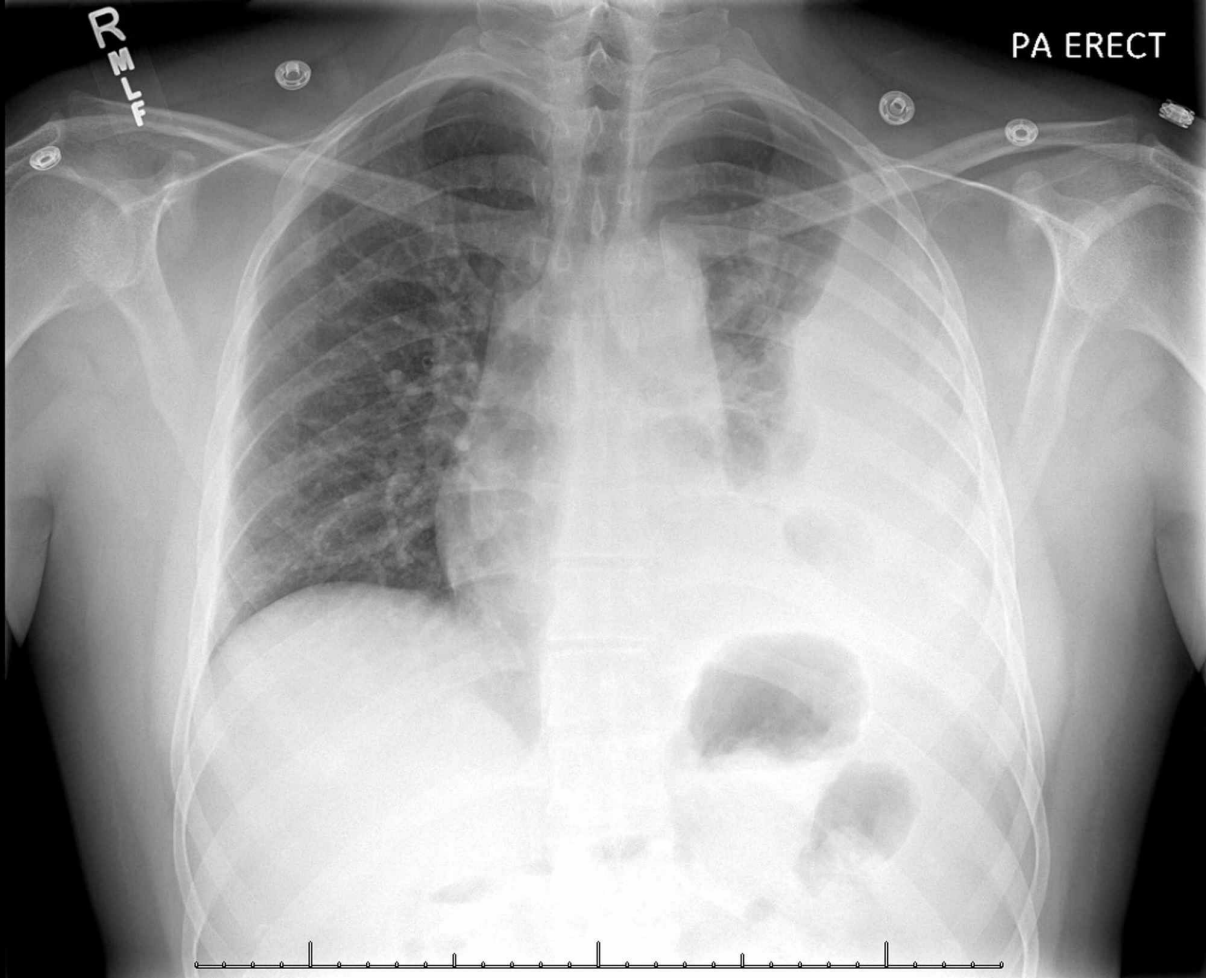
Initial chest x-ray of the patient showing left-sided pleural effusion with atelectasis.
